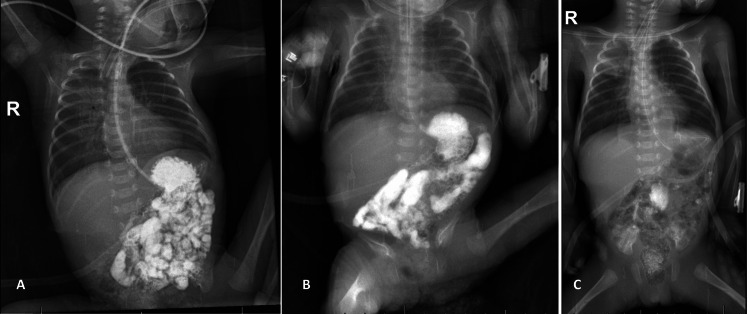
Sequential X-ray images with oral contrast medium showing the progression of contrast through the gastrointestinal tract The series includes images at (A) two hours, (B) six hours, and (C) 24 hours after ingestion, demonstrating unobstructed flow into the rectum and beyond without any spillage or obstruction.
